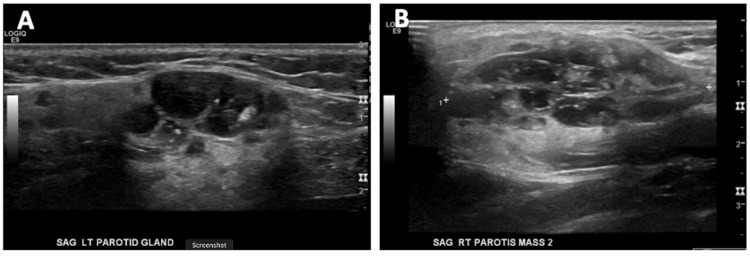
Sagittal ultrasound of the left (A) and right (B) parotid glands depicting bilateral complex solid/cystic intraparotid gland masses
